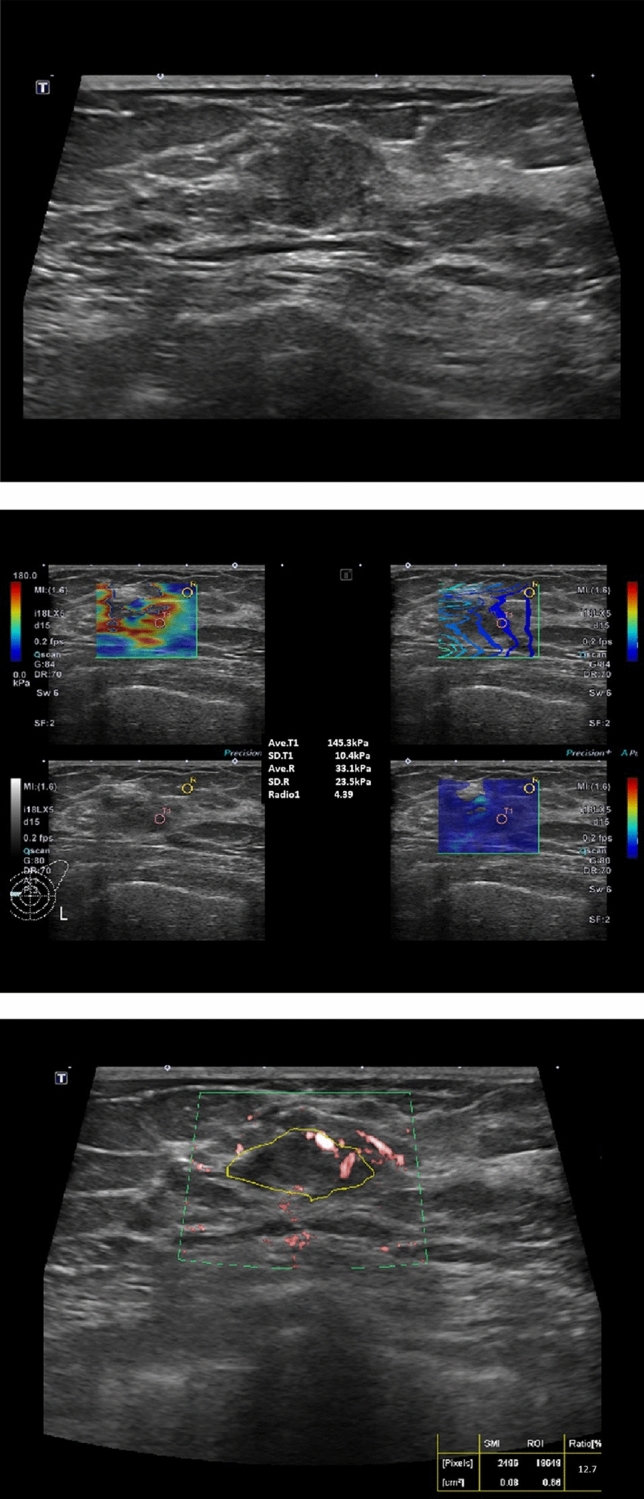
A 79-year-old woman with proven invasive ductal carcinoma. (A) B-mode ultrasound was a 1.5 cm-sized mass categorized as C4a. (B) The SWEmax was 145.3 kPa and the SWE ratio was 4.39. (C) The SMI vascular index was 12.7% over the cutoff value. The combined score was 4, indicating that the lesion was considered malignant lesion and the pathologic diagnosis was IDC grade I with DCIS.
